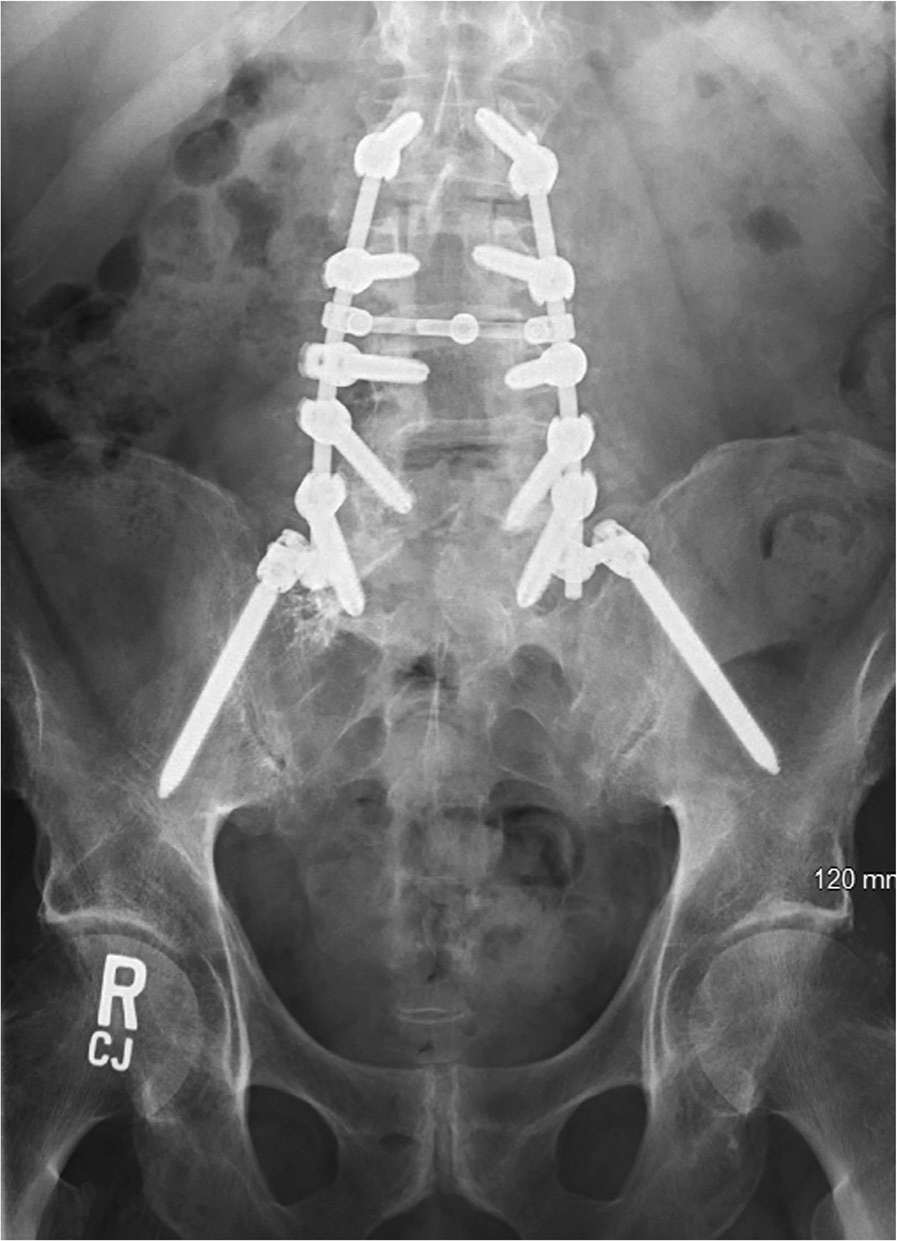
AP Lumbar X-ray: L2-Pelvis fusion construct
